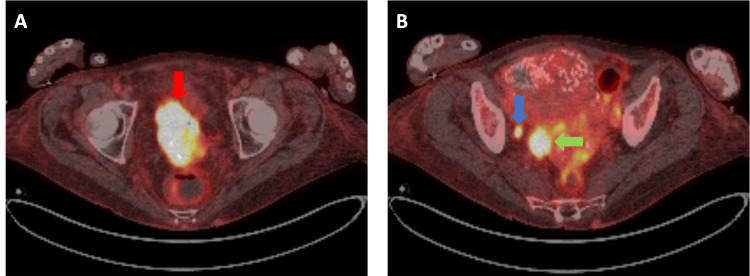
Pre-treatment PET-CT This patient had IVA disease, including a bulky primary tumor invading the bladder (red arrow, panel A) as well as pelvic node (blue arrow, panel B) and right ovarian (green arrow, panel B) metastases, amongst other clinical findings. PET-CT: Positron emission tomography-computed tomography
